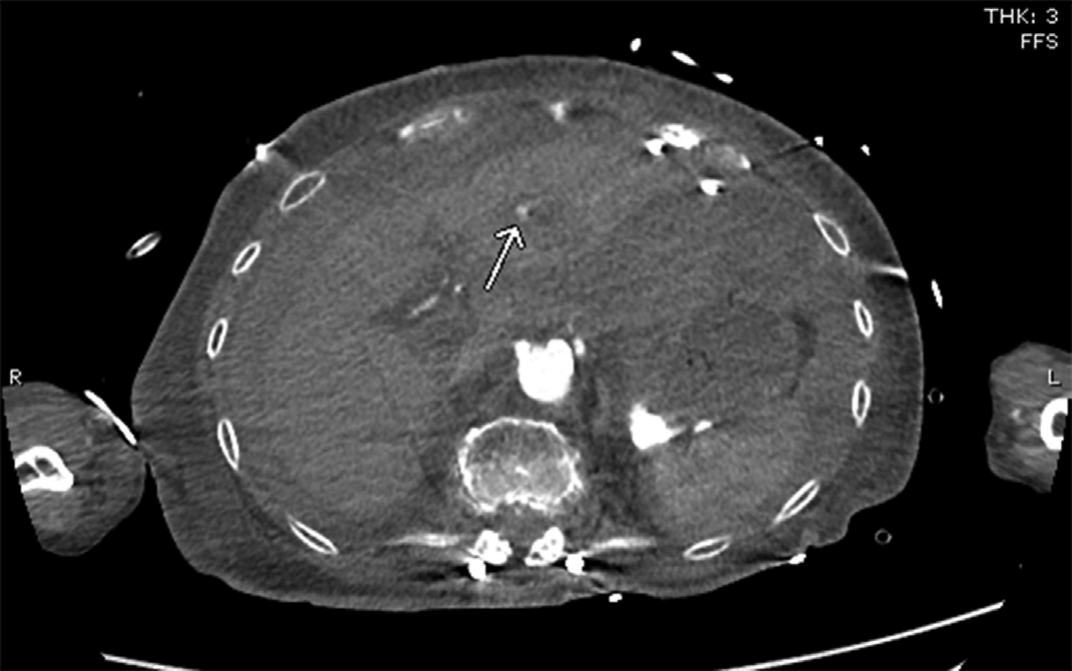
Computed Tomography Angiogram With Intravenous Contrast Material Arrow points to the adjacent hyperenhancement on arterial phase representing shunting.
